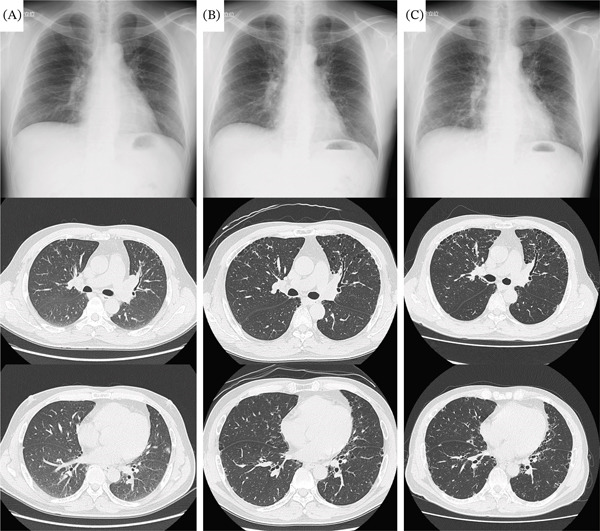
Comparison of chest radiography and high‐resolution computed tomography (HRCT) imaging prior to referral to our hospital. Progression of linear opacities, reticulation and traction bronchiectasis is evident. (A) Thirteen years before referral—2 years after the patient underwent whole lung lavage procedures. (B) Two years before referral. (C) Four months before referral.
